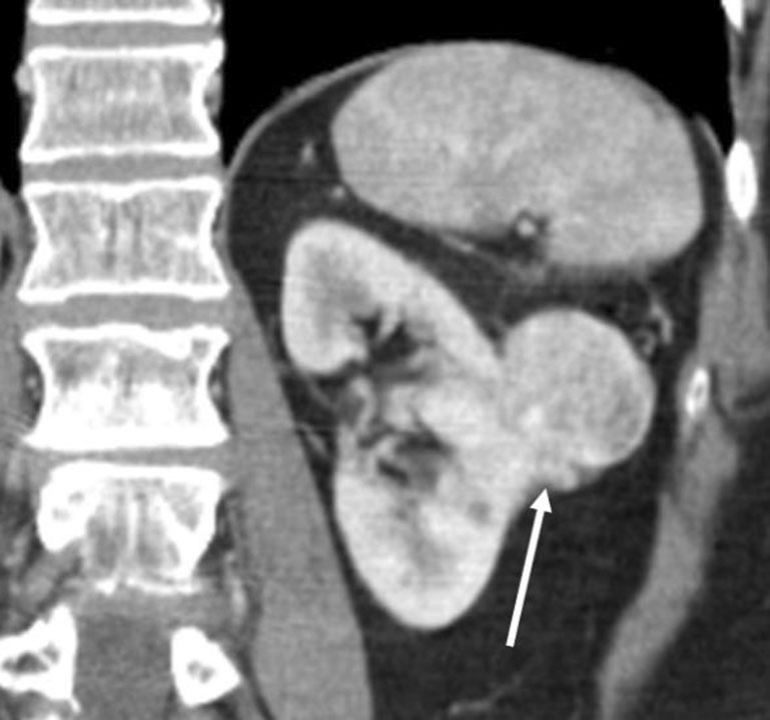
A 75-year-old woman with a left renal oncocytoma with perinephric fat extension. The coronal reformatted image of the portal venous phase of CE-CT shows tumour extension into the perinephric fat at the inferior aspect of the tumour (*arrow*)
